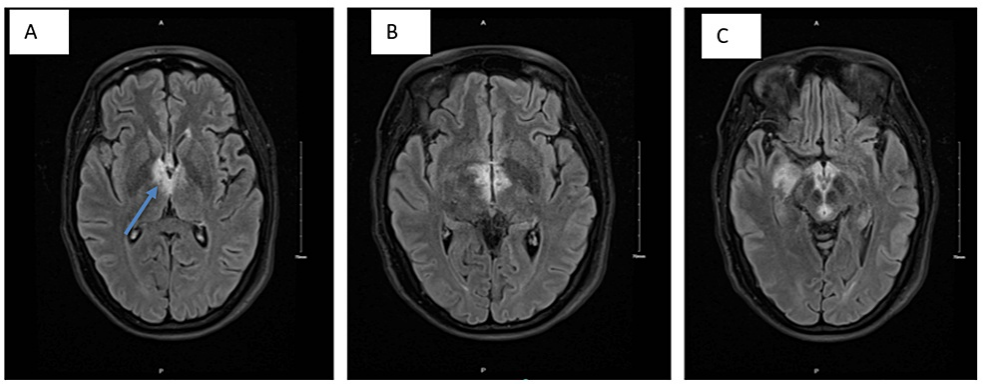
A, B, C: MRI brain T2-FLAIR axial view with and without IV contrast. (a) Hyperintensity around the third ventricle, with slightly increased prominence on the right. (b) Hyperintensity around the third ventricle, with slightly increased prominence on the right. (c) Hyperintensities involving the bilateral mamillary bodies, periaqueductal grey/tectal plate, around the third ventricle, anterior to the fourth ventricle, bilateral anteromedial thalami, right greater than left, interthalamic adhesion, right parahippocampal gyrus anterior to the right temporal horn
